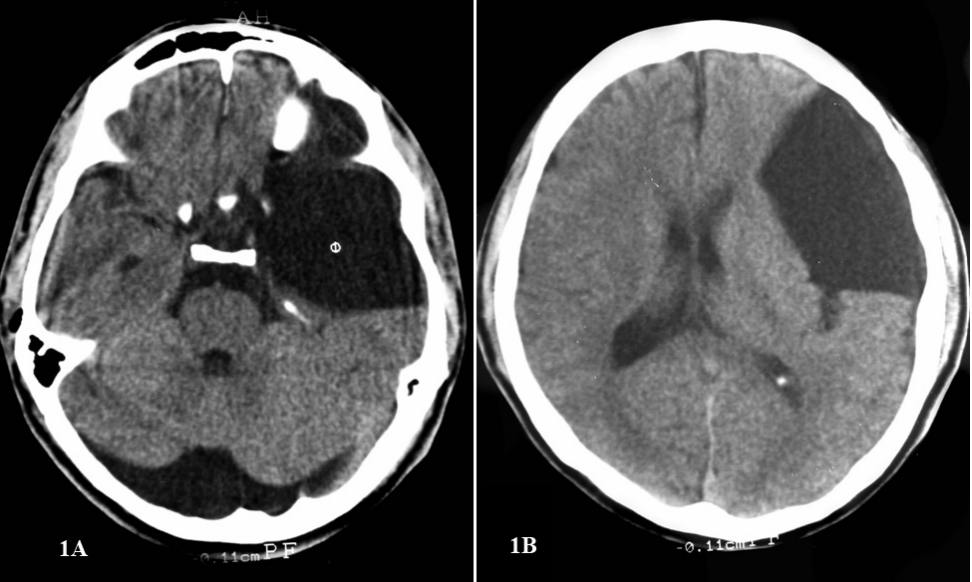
Computed tomographic scan of the head without contrast immediately following the trauma, showing a giant arachnoid cyst in the middle cranial fossa.
